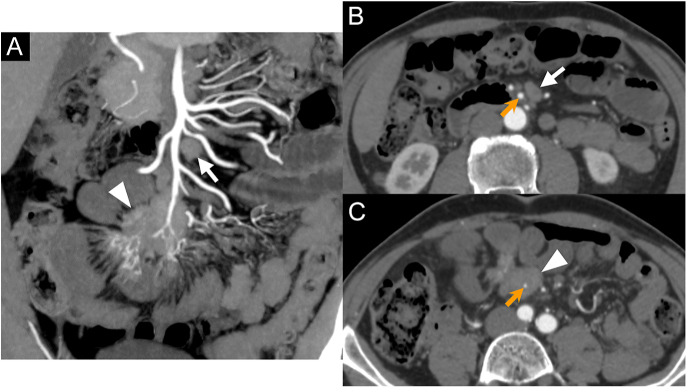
How to distinguish mesenteric lymph nodes metastases and mesenteric tumor deposits. Images A, B and C are from the same CT (arterial phase) in a 69-year-old man. (A) Coronal MIP 10 mm reconstruction at the arterial phase showing mesenteric mass (white arrowhead) involving the distal part of the SMA trunk and mesenteric lymphadenopathy (white arrow) located high up in the mesentery, close to the first jejunal branches. (B) Axial MPR 1 mm reconstruction at the level of the mesenteric lymphadenopathy (white arrow), which is close to the trunk of the SMA (orange arrow), but there is no contact (persistent fat plane between them). (C) Axial MPR 1 mm reconstruction at the level of the mesenteric mass (white arrowhead), which encases (contact with >180°) the trunk of the SMA (orange arrow).
